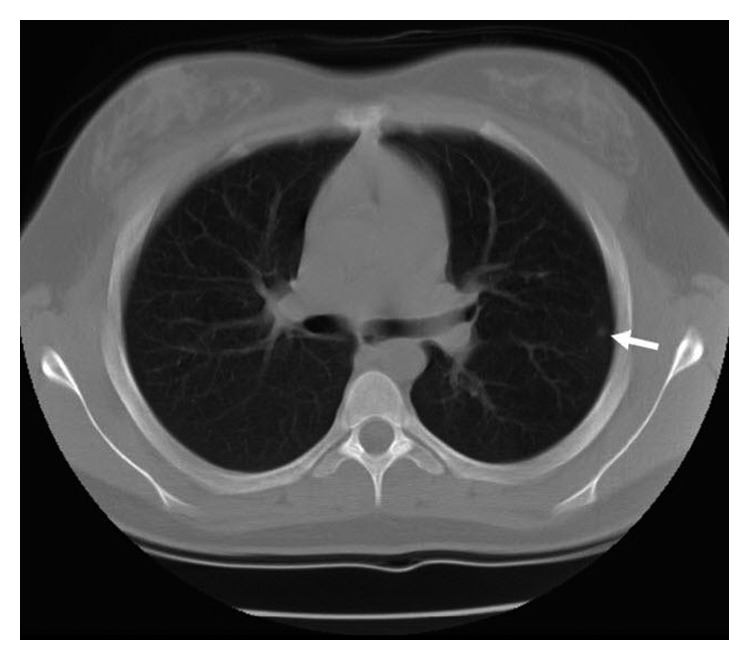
An axial computed tomography (CT) scan of the chest demonstrating a subpleural lesion (white arrow) identified at the time the adamantinoma was originally diagnosed. The lesion was biopsied due to concerns of metastasis, and the biopsy revealed benign fibrous lung tissue.
